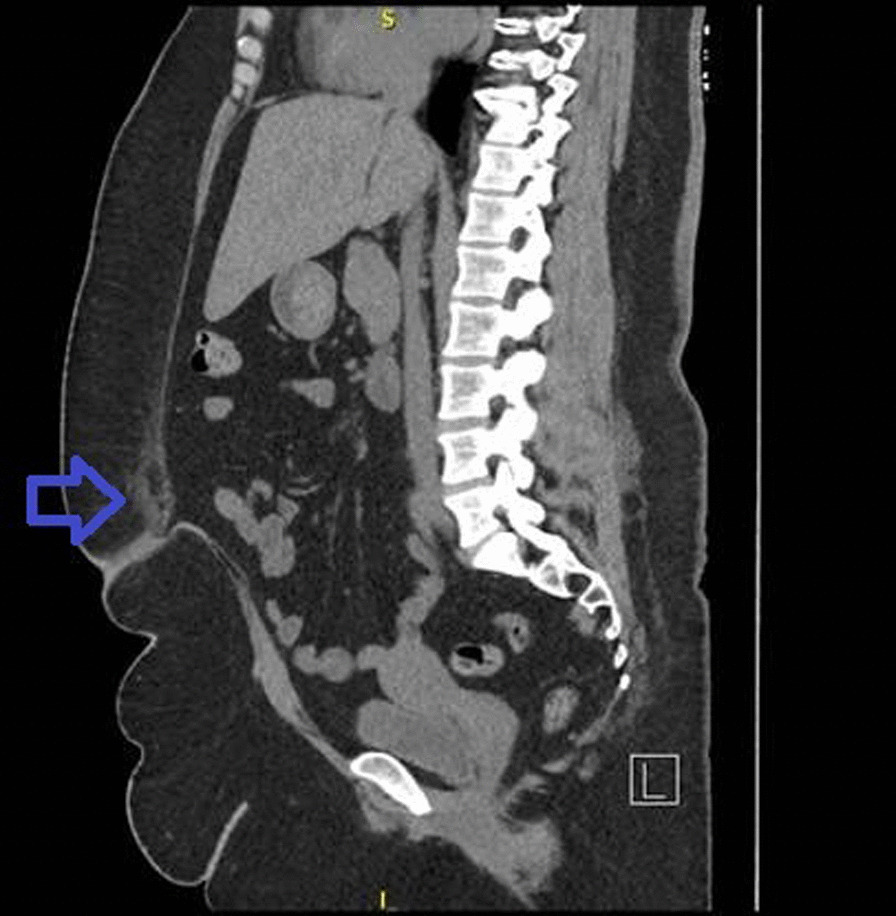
Sagittal computed tomography of the abdomen revealing an umbilical hernia (blue arrow) with fat superior to the umbilicus and hernia
